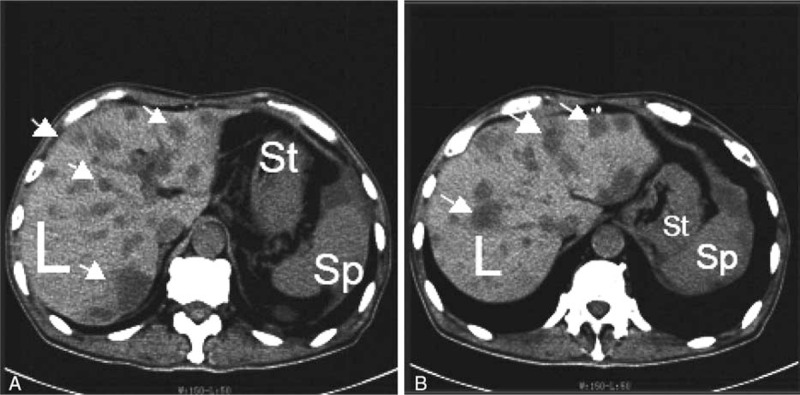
CT Scan of patient on second admission. (A & B) Presence of multiple liver lesions (arrows). L: Liver; Sp: Spleen; St: Stomach.
